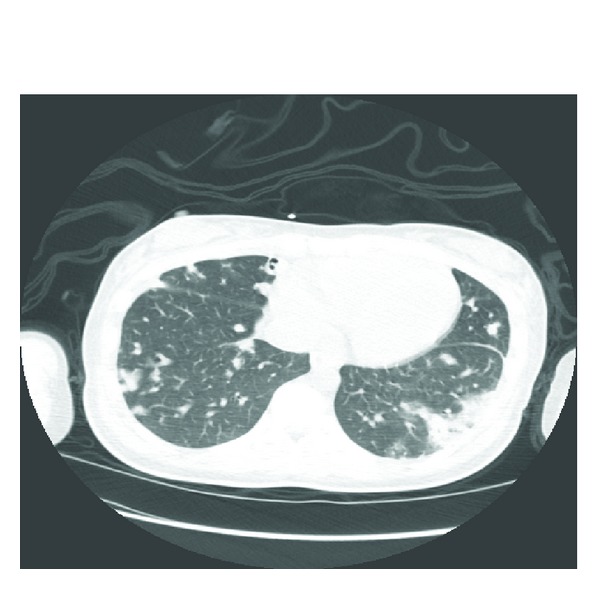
Chest CT scan showing pulmonary nodules.
